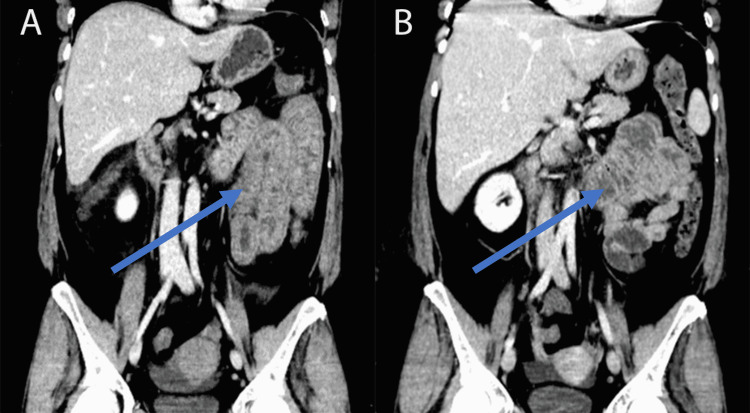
CT abdomen and pelvis with contrast (A) on day of admission and (B) nine days later, showing some improvement of pancolitis (blue arrow).
